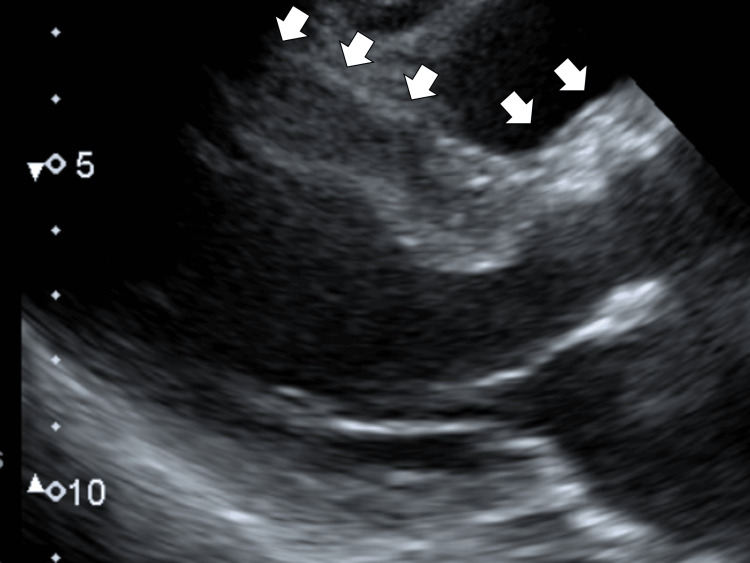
Initial echocardiography showing left ventricular septum thickening without the narrowing aortic valve region (white arrows)
